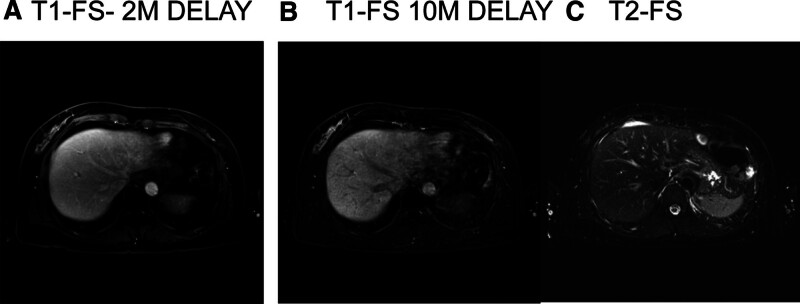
Follow-up MRI performed 2 mo after the radiation-induced liver disease diagnosis. FS = fat suppressed imaging, M = minutes, MRI = magnetic resonance imaging.
